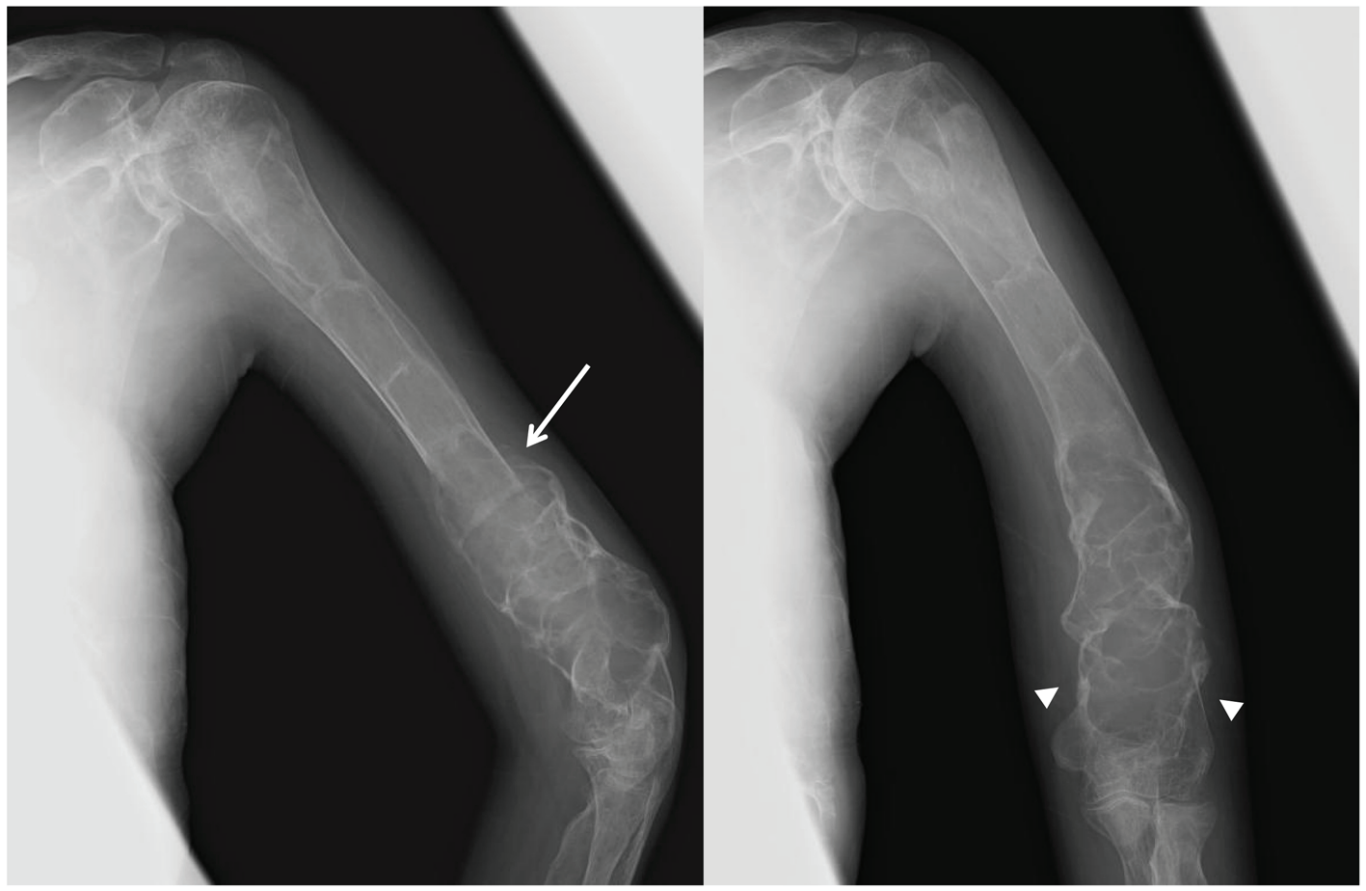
X-ray images of the left humerus after 1 year from the zoledronate administration. Radiological thickening of the humeral shaft cortical bone, progressive ossification in the fibrodysplastic lesion, and periosteal bone formation (arrow) are observed. Fracture lines have completely disappeared (arrow head).
